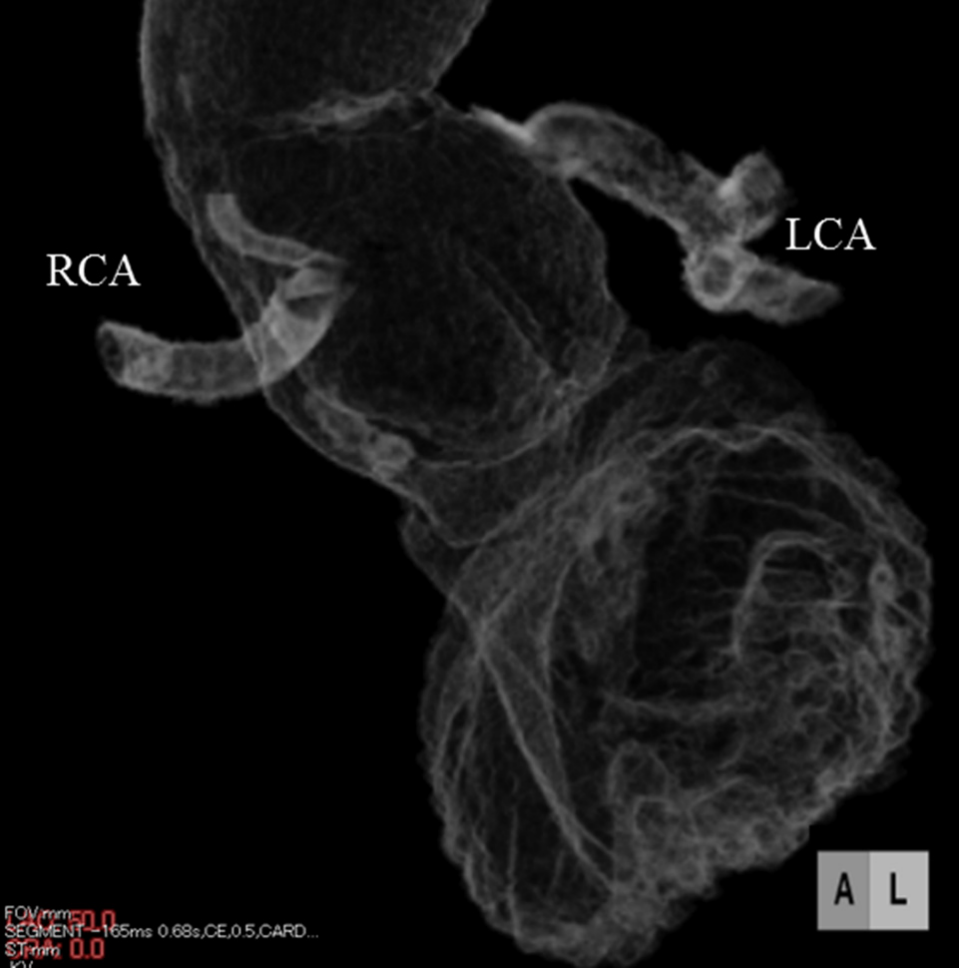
Postoperative 3-dimensional computed tomography of the aortic root revealed no residual pseudoaneurysm. *RCA*, Right coronary artery; *LCA*, left coronary artery.
